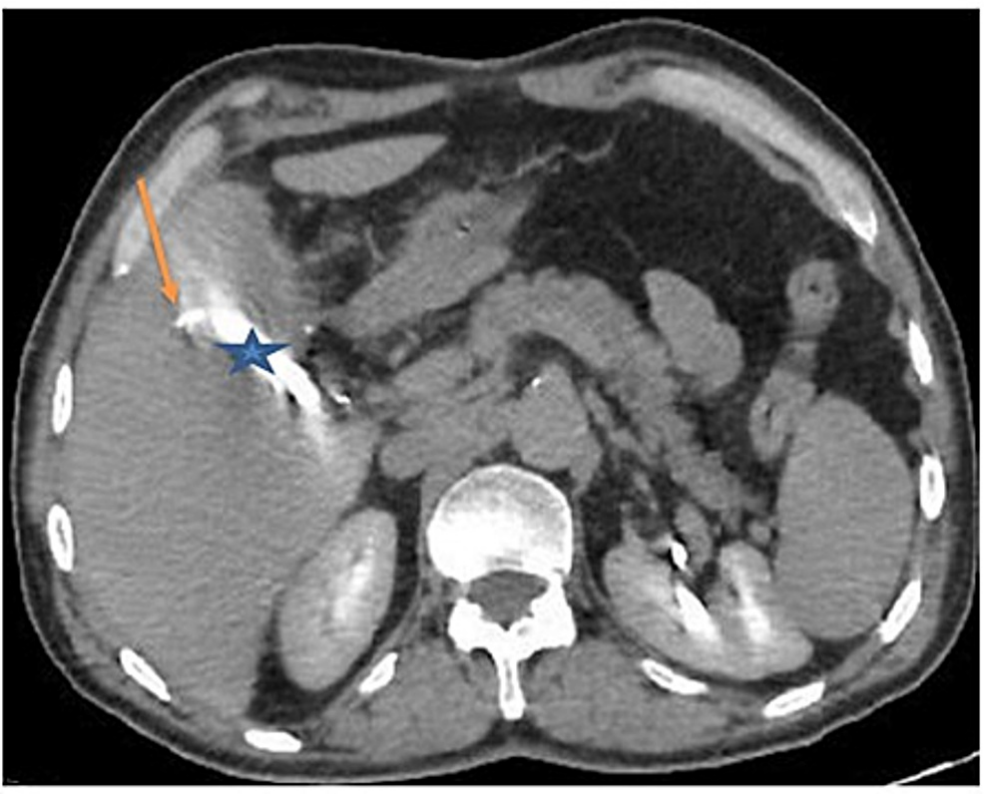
CT scan after drain opacification - view 1 The image shows opacification of the vesicular bed (blue star) and a small intrahepatic bile duct at segment V, which is connected to the injured duct of Luschka (orange arrow) CT: computed tomography
